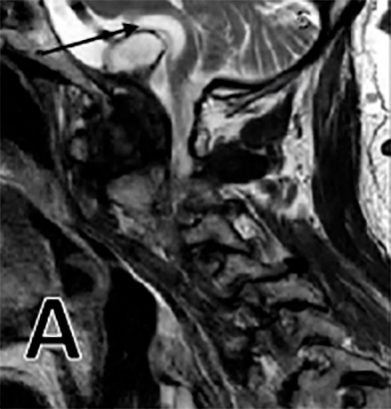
Pre-operative MRI sagittal T2 sequence, hyperintense signal (black arrow) within medulla representing encephalomalacia due to pannus compression.
